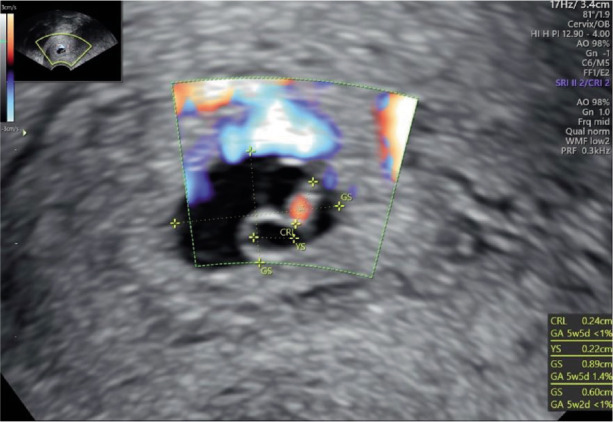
Doppler; blood flow surrounding gestational sac.
